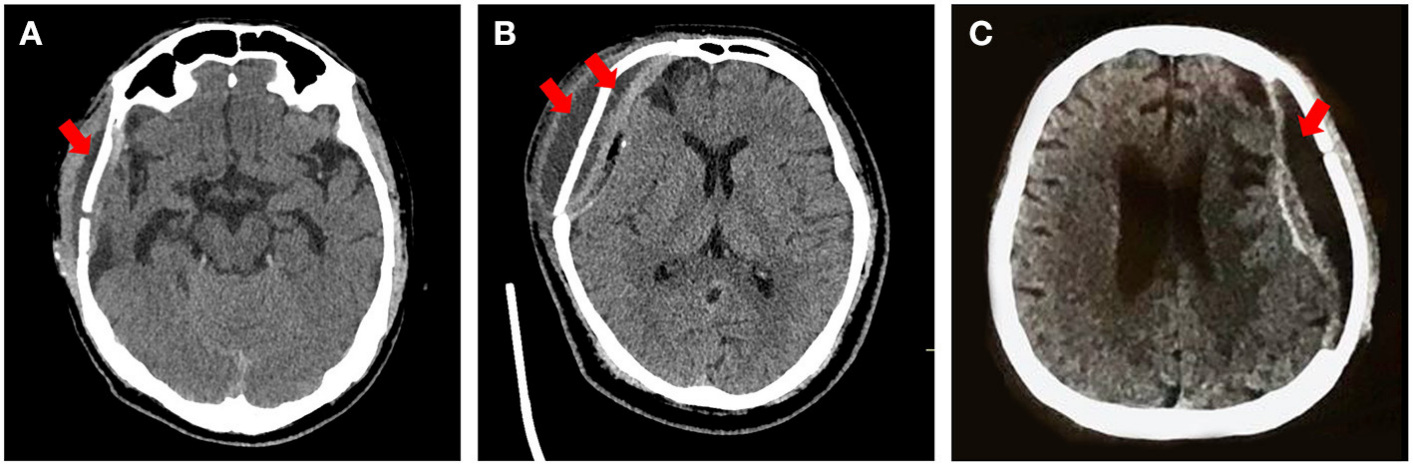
Typical types of effusion after PEEK cranioplasty. Red arrow: the effusion presents as a low-density area under the scalp on CT. (A) Subcutaneous effusion mainly outside the plate. (B) Subcutaneous effusion complicated with epidural effusion. (C) Effusion only at the epidural space.
